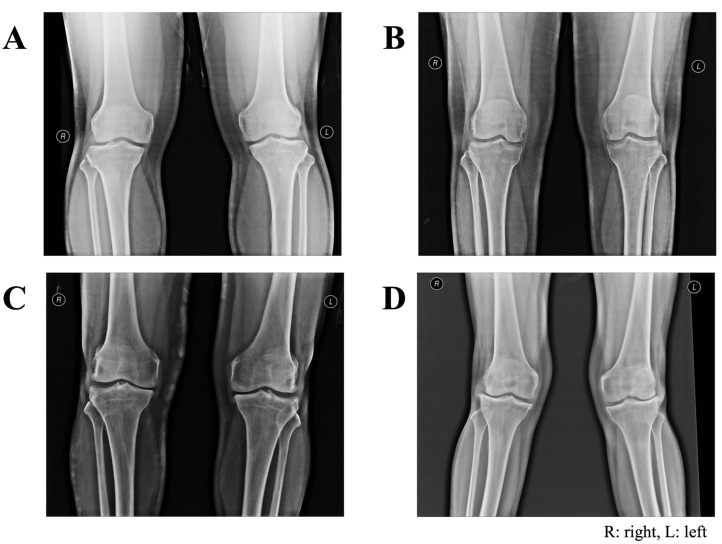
Examples of anteroposterior radiographs of paired knees presented in the current study: (A) bilateral KL grade 0, demonstrating normal bone density and good alignment; (B) bilateral KL grade 1, demonstrating possible joint space narrowing with small osteophytes in right knee; (C) bilateral KL grade 2, demonstrating narrow knees and patellofemoral joints with spur; (D) bilateral KL grade 3, demonstrating mild genu varus deformity with no displaced fracture, subchondral sclerosis with joint space narrowing, and osteophyte formation in lateral tibiofemoral joint space.
